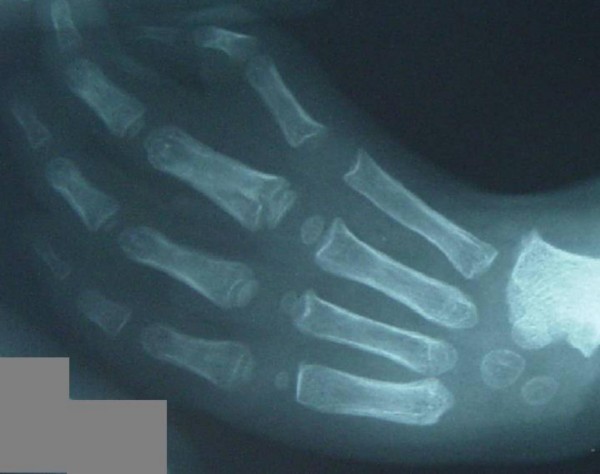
Anteroposterior radiograph of the hand showed the absent thumb.
